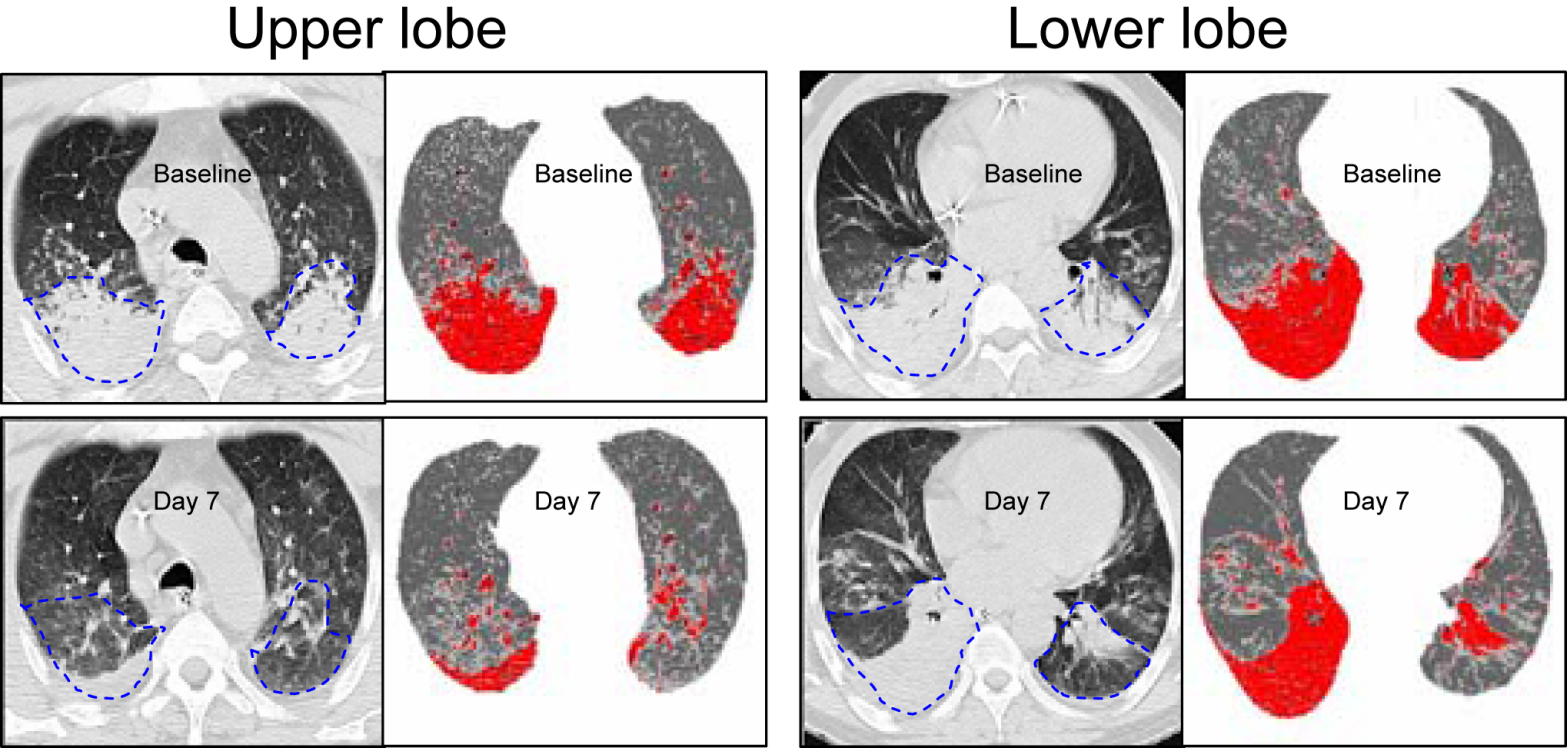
Representative CT sections of upper and lower lobes obtained at baseline and day 7 in a patient with acute respiratory distress syndrome. Computed tomography (CT) sections at baseline and day 7 are at the same lung region as attested by the anatomical landmarks present on the rough images at baseline and day 7 (aortic arch and vascular divisions for upper lobe CT sections and vascular divisions for the lower lobe CT sections). As previously described [18], poorly and nonaerated lung areas of right and left upper and lower lobes are manually delineated (dashed line) at baseline (before HL-10 administration) with the aid of the software Lungview) that identifies poorly and nonaerated lung areas in light gray and red, respectively. Delineation performed at baseline is manually 'transposed' to the CT section corresponding to the same anatomical level obtained at day 7. Surfactant-induced lung reaeration is defined as the increase in gas volume within the delineated zone between day 7 and baseline. The same process is repeated on each CT section in order to assess overall surfactant-induced lung reaeration.
